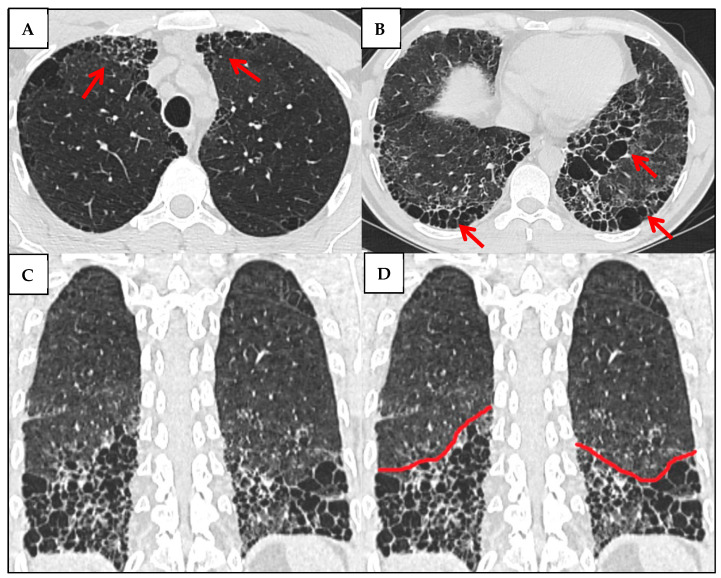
HRCT Scans in a 35-Year-Old Patient with Rheumatoid Arthritis and ILD with an UIP Pattern. Image (A) demonstrates the “upper lobe sign,” characterized by predominant fibrotic involvement of the anterior segments of the upper lobes (red arrows). Image (B) shows “exuberant honeycombing,” with extensive, clustered cystic airspaces comprising more than 70% of the fibrotic regions (red arrows). Coronal reconstructions (Images C,D) reveal the “straight edge sign,” particularly evident in Image D with the red demarcation line, indicating an abrupt interface between normal and fibrotic lung tissue, forming a well-defined linear boundary.
